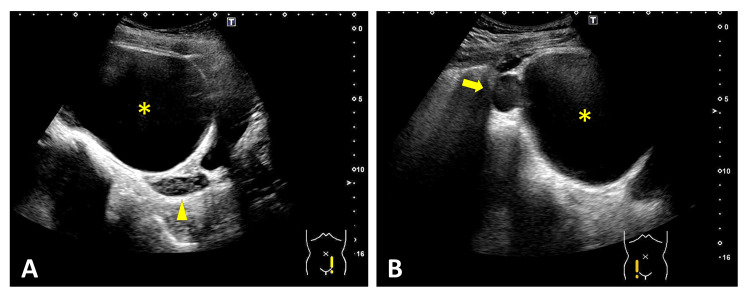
Double-Bladder Sign on RADUS Longitudinal RADUS (A) demonstrated a cystic mass (*) producing the double-bladder sign, with the normal left ovary (arrowhead) identified posterior to the cystic lesion. A mass with a daughter cyst sign was seen superior to the lesion on the right side (B, arrow). RADUS: radiology-performed ultrasound
